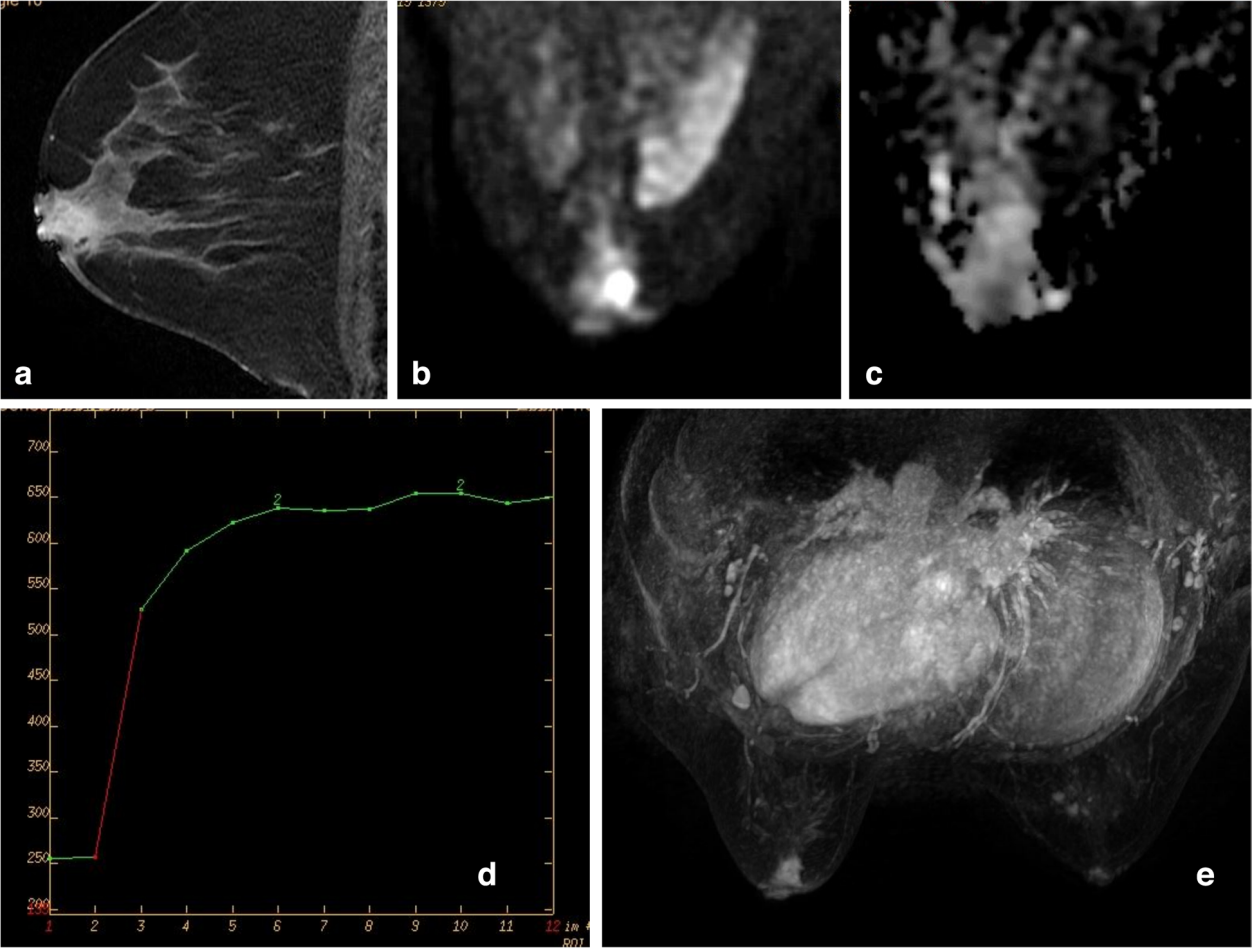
a A lesion in a 39-year-old female with focal and heterogeneous enhancement in theleft breast. b and c The ADC value was 0.93 × 10^–3^ mm^2^/s. d The imaging revealed a type TICII curve. e No increasing in vasculature. The multiparametric score was 2, the lesion was determined as BI-RADS-MRI type 4a. The pathological finding was inflammation with abscess formation
